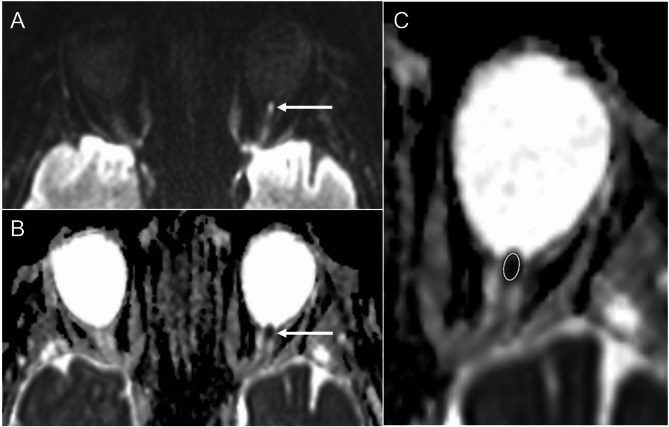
Qualitative and quantitative ADC map assessment in a patient with left anterior ischemic optic neuropathy. Hyperintensity of the left optic nerve head is visible on DWI (A). Corresponding reduction of the ADC inferior to the signal of the white matter of the temporal lobe is visible (qualitative assessment, (B). For the quantitative assessment, a region of interest (ROI) presenting the lowest signal on ADC maps was placed within the optic nerve head/intraorbital portion of the optic nerve (C)
